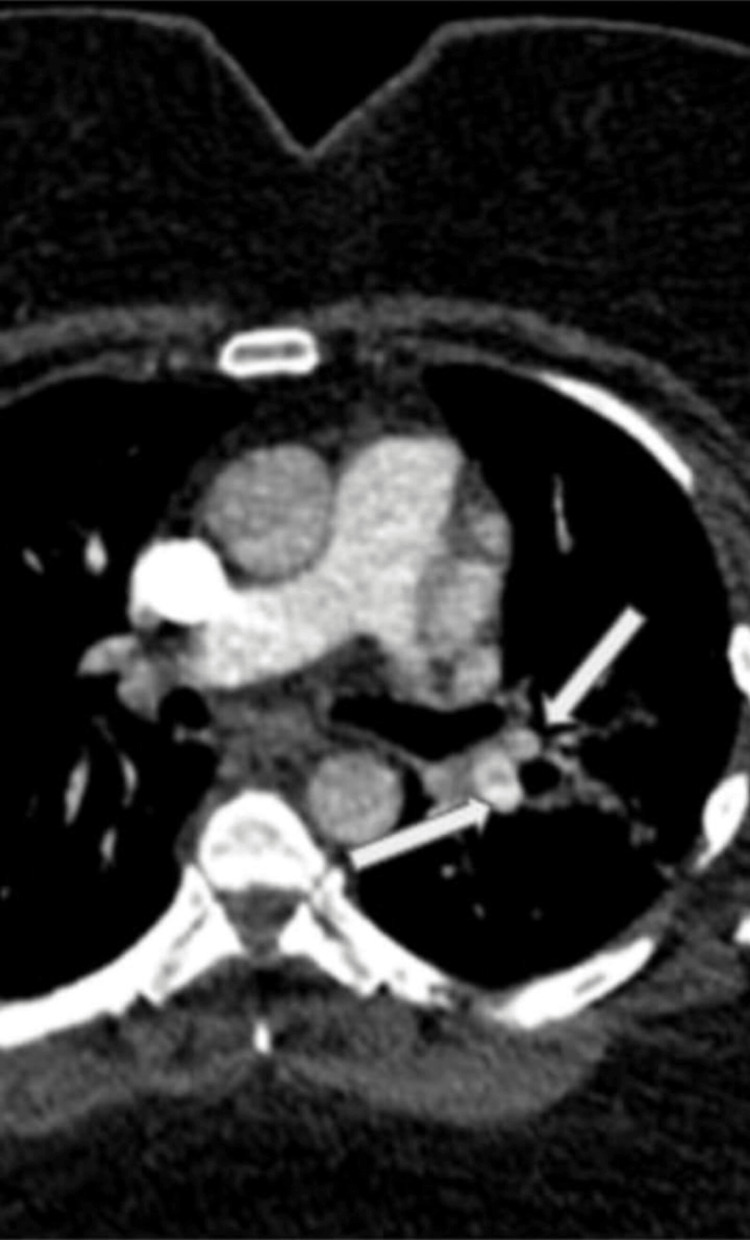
Zoomed-up axial CT pulmonary angiogram section showing partial filling defects within the left lower lobe and lingular segment branches
